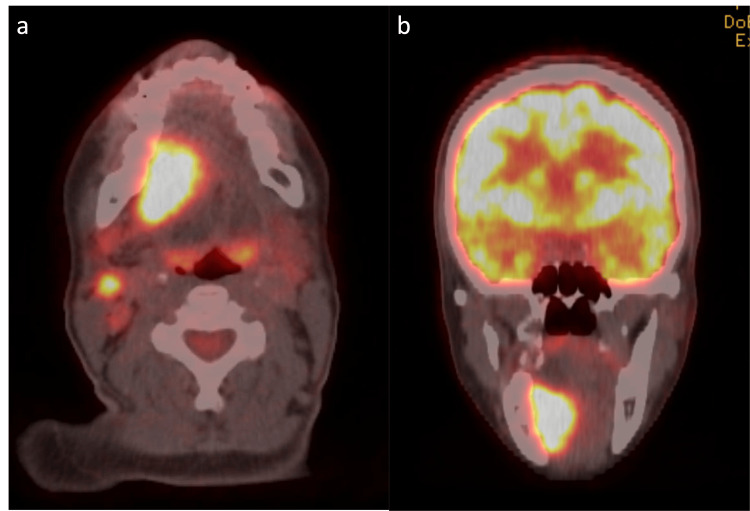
Axial (a) and coronal (b) PET-CT scans showing hypermetabolic activity in the right floor of the mouth and a metastatic lymph node consistent with biopsy-proven malignancy PET-CT: positron emission tomography-computed tomography
